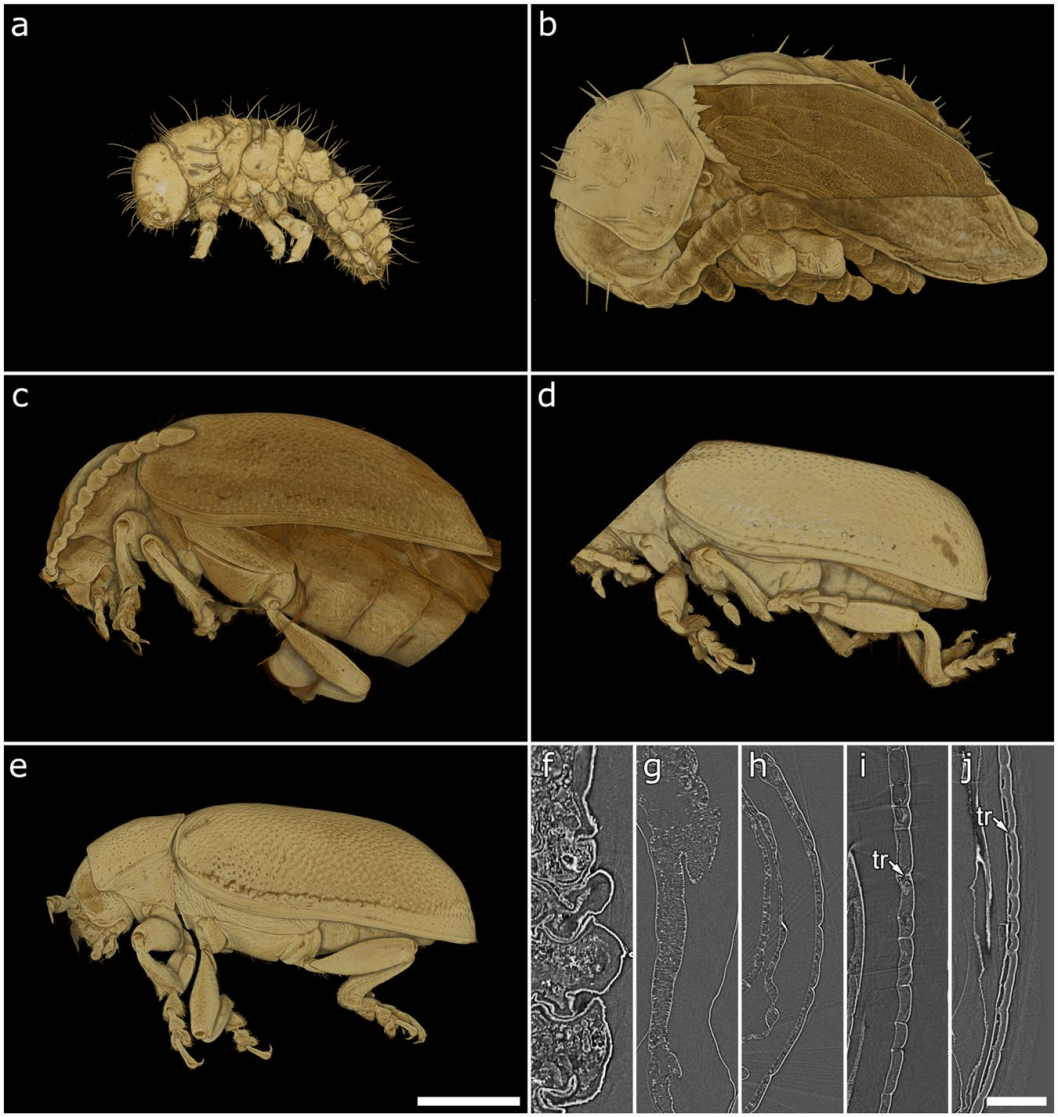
Results from SR-*μ*CT scans showing volume renderings (a–e) and cross-sections (f–j) of the respective dataset. 3rd instar larva, which exhibits a soft cuticle (a,f); young pupa (b,g), pupal skin partly digitally removed to reveal the developing elytron; imago immediately after ecdysis (c,h) the young imago (d,i), which shows a fully-formed yet rucked up cuticle and the formation of trabeculae (“tr” in the figure); old imago (e,j) with a fully developed cuticle. Scale bars: 1 mm.
